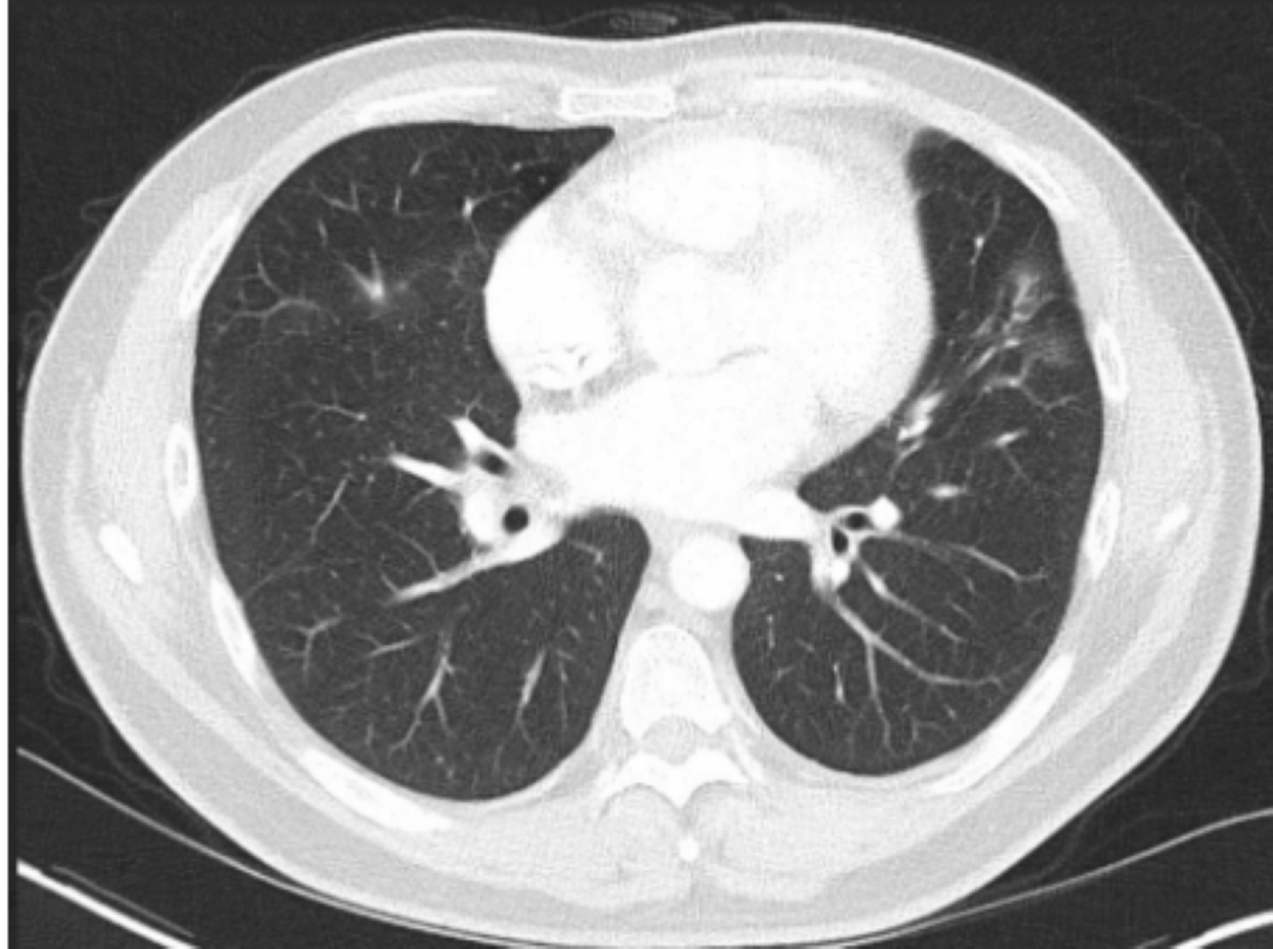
Chest computed tomography (CT) scan at the 18-month follow-up demonstrates marked improvement in the cavitary lesion and significant resolution of lymphadenopathy.
